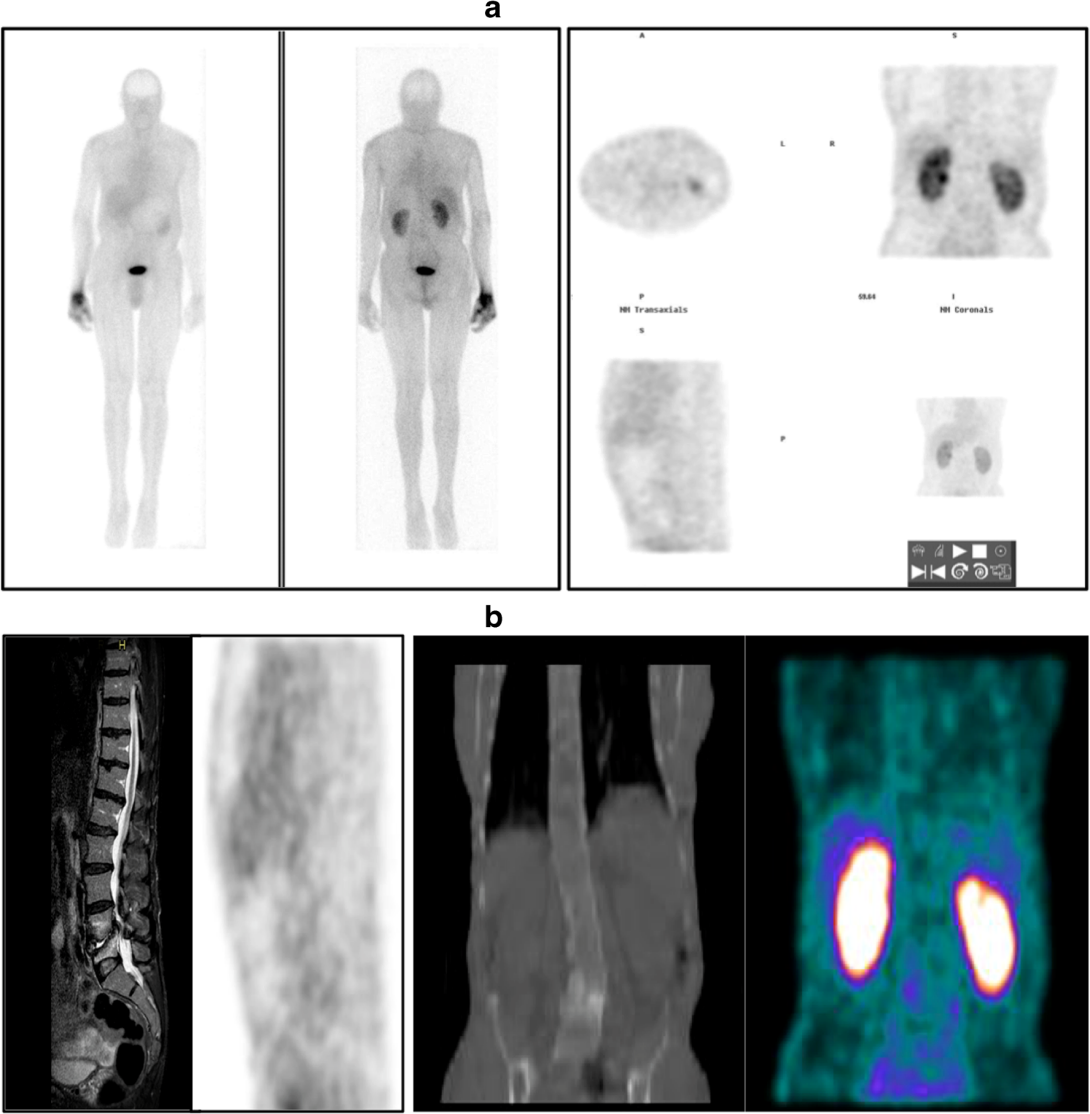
A 57-year-old man presented with back pain, fever, and raised CRP level (21.32 mg/L) about 12 months after being submitted to lumbar surgery L3-L5. (a): anterior and posterior whole-body views recorded about 30 min after injection of ^99m^Tc-UBI 29-41 (left panel), and selected tomographic sections of the emission SPECT/CT scan acquired immediately thereafter (right panel). (b), from left to right: sagittal MRI section, sagittal SPECT section, coronal CT section, and coronal fused SPECT/CT section. MRI demonstrates enhancement of end plates and disc indicating active infection. Whereas, no clear foci of abnormal ^99m^Tc-UBI 29-41 uptake can be detected in either the planar or SPECT/CT images; physiologic tracer uptake in the liver of this patient appears lower than in the patients of Figs. 1 and 2 because the images are displayed with lower contrast. Following prolonged high-dose antibiotic therapy all symptoms disappeared and the serum CRP level returned to normal. This case was therefore classified as a false negative ^99m^Tc-UBI 29-41 SPECT/CT scan
